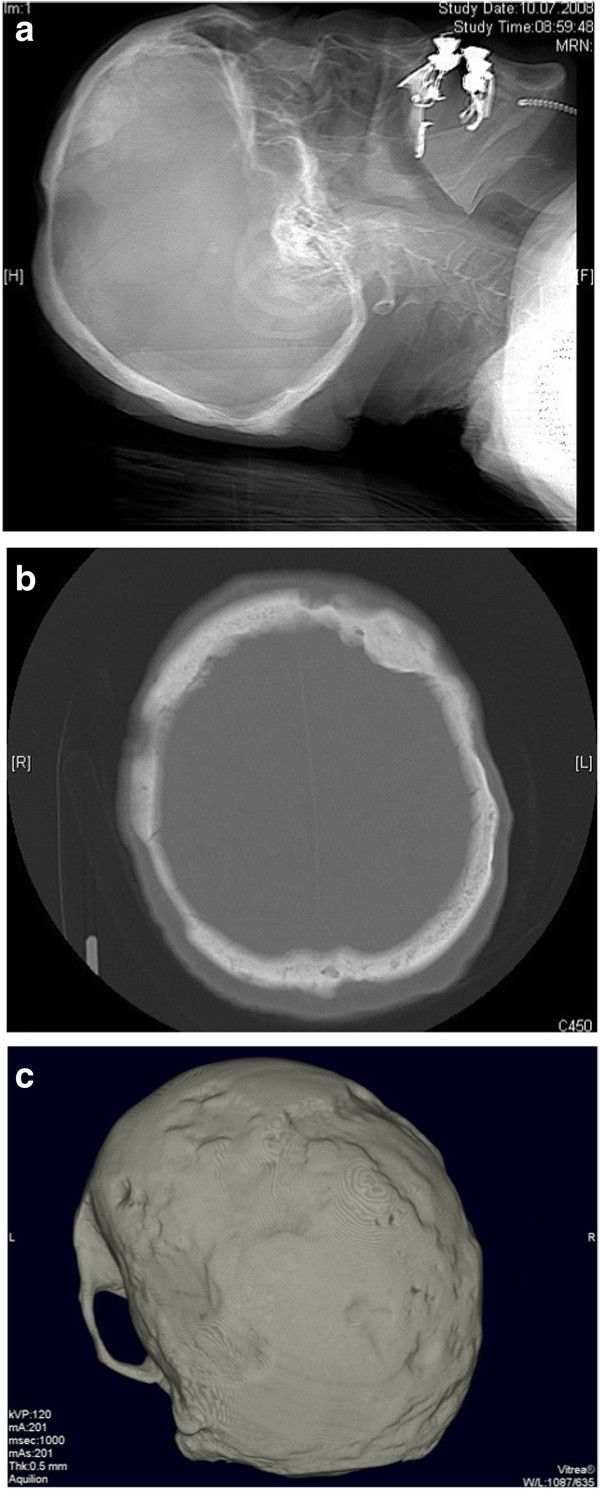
Pronounced recalcification of the skull bone four months after radiation treatment with a total dose of 10 Gy in: a) lateral radiograph b) transversal CT scan (bone window) c) 3D-CT reconstruction.
